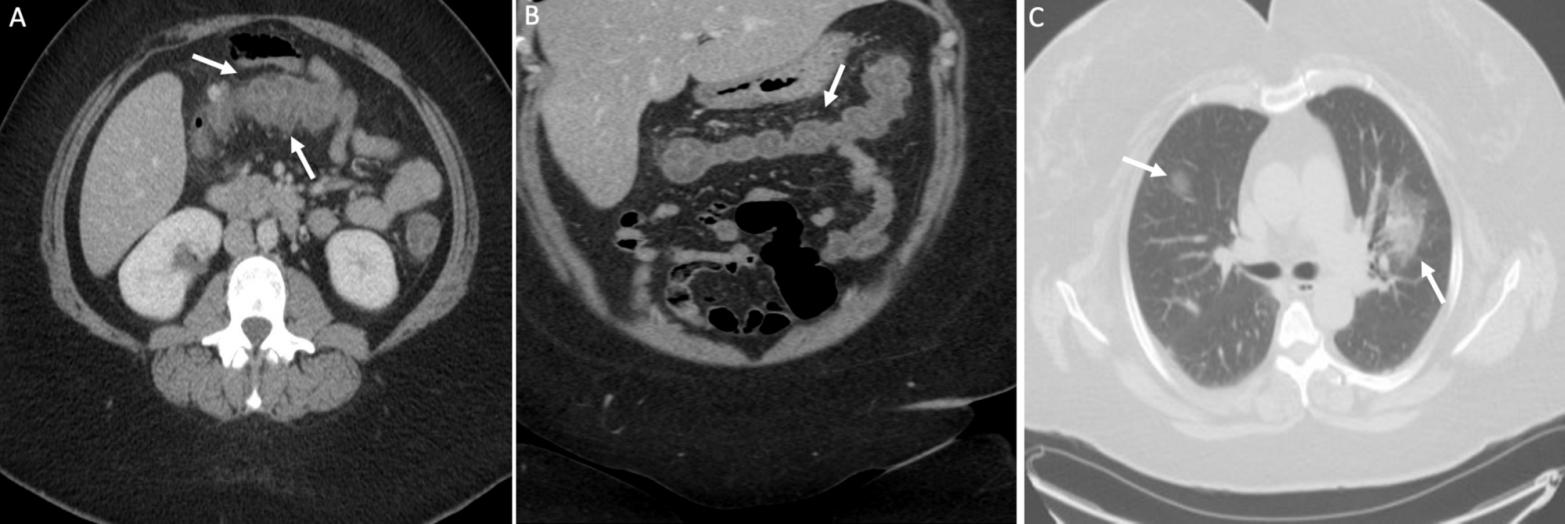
CT showing acute colitis Axial (A), coronal (B), and axial (C) CT of the abdomen and pelvis with IV contrast of a 24-year-old woman with abdominal pain and diarrhea. The edematous appearance of the transverse colon (arrows) with surrounding hyperemia is compatible with acute colitis. Lung findings in image C show typical COVID-19-associated bilateral nodular ground-glass opacities (arrows) CT: computed tomography; IV: intravenous; COVID-19: coronavirus disease 2019
